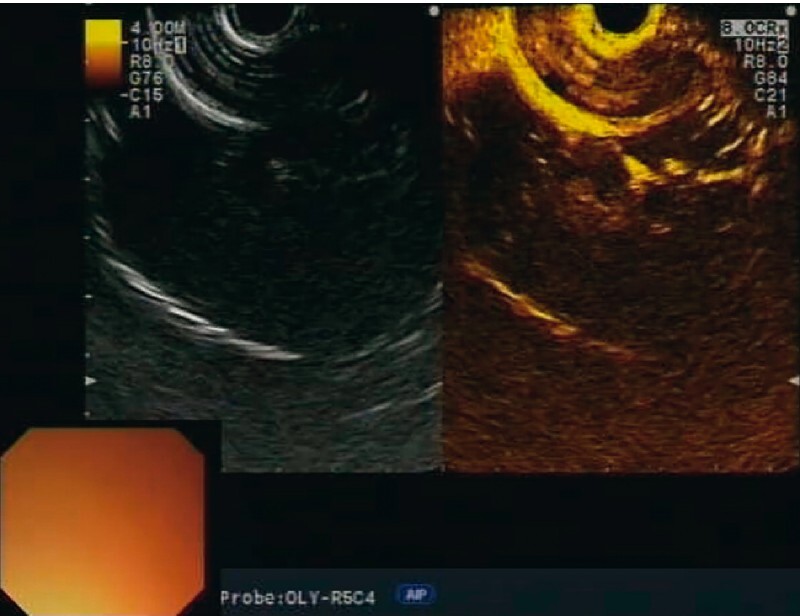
Endoscopic ultrasound view of the lesion showing a diffuse and inhomogeneous hypo-enhancing pattern following contrast injection.
